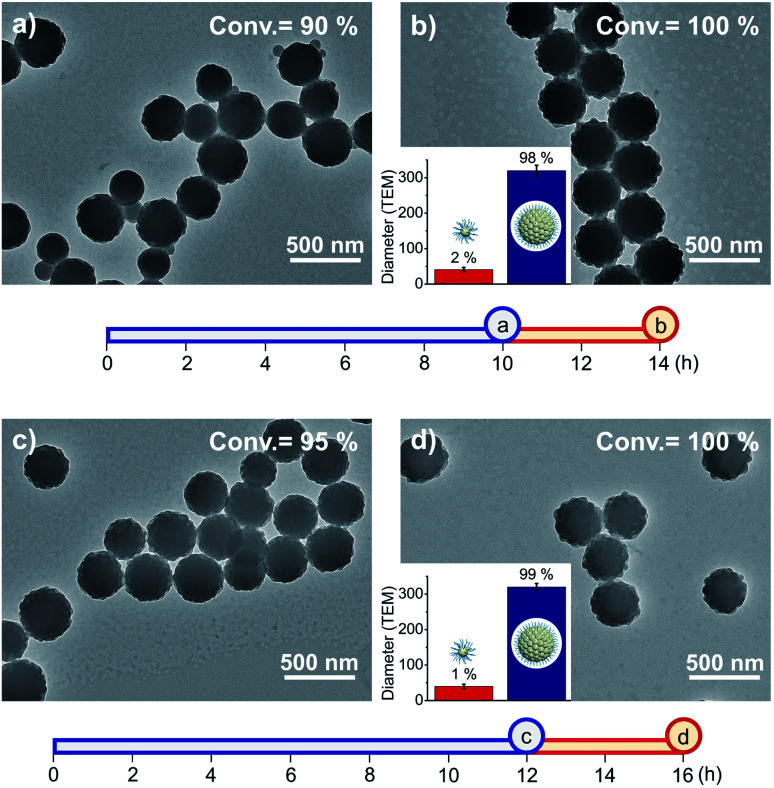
TEM images of fluorinated particles prepared by successive photo-RDRP using two light intensities. [PFS]/[PDMA_54_-CTA]/[PC] = 200 : 1 : 0.1. (a and c) Reaction mixtures were exposed to weak-light irradiation for 10 and 12 h, respectively. (b and d) Strong-light irradiation (4 h) was subsequently used following (a) and (c). The size and distribution of particles depicted in Fig. 2b and d were obtained by analysing approximately 400 random particles. The two axes exhibit the exposure times of two light intensities: blue colour for 0.56 mW cm^−2^, red colour for 33 mW cm^−2^.
